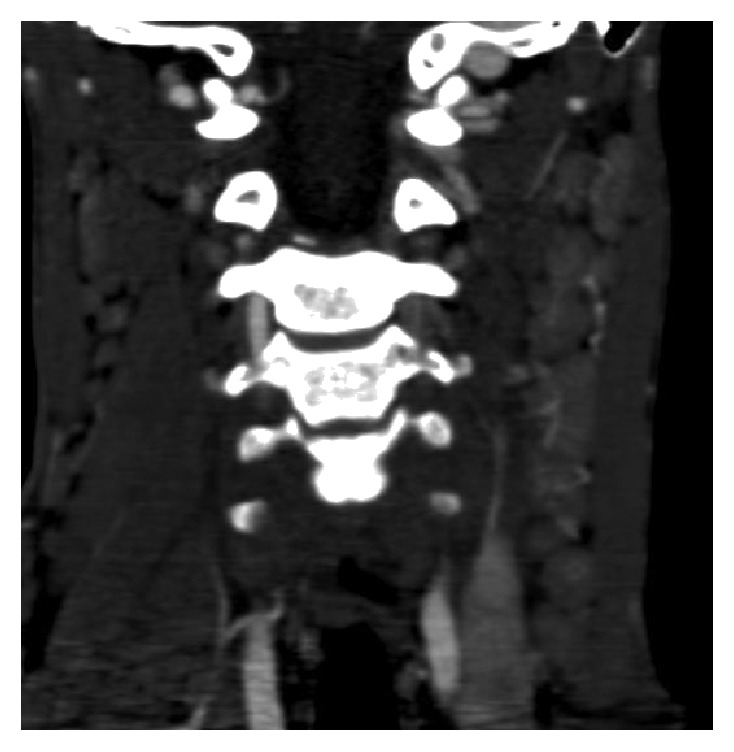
Coronal CT angiogram of the neck demonstrating extensive hypervascularity of cervical lymph nodes bilaterally which are more pronounced on the left side.
